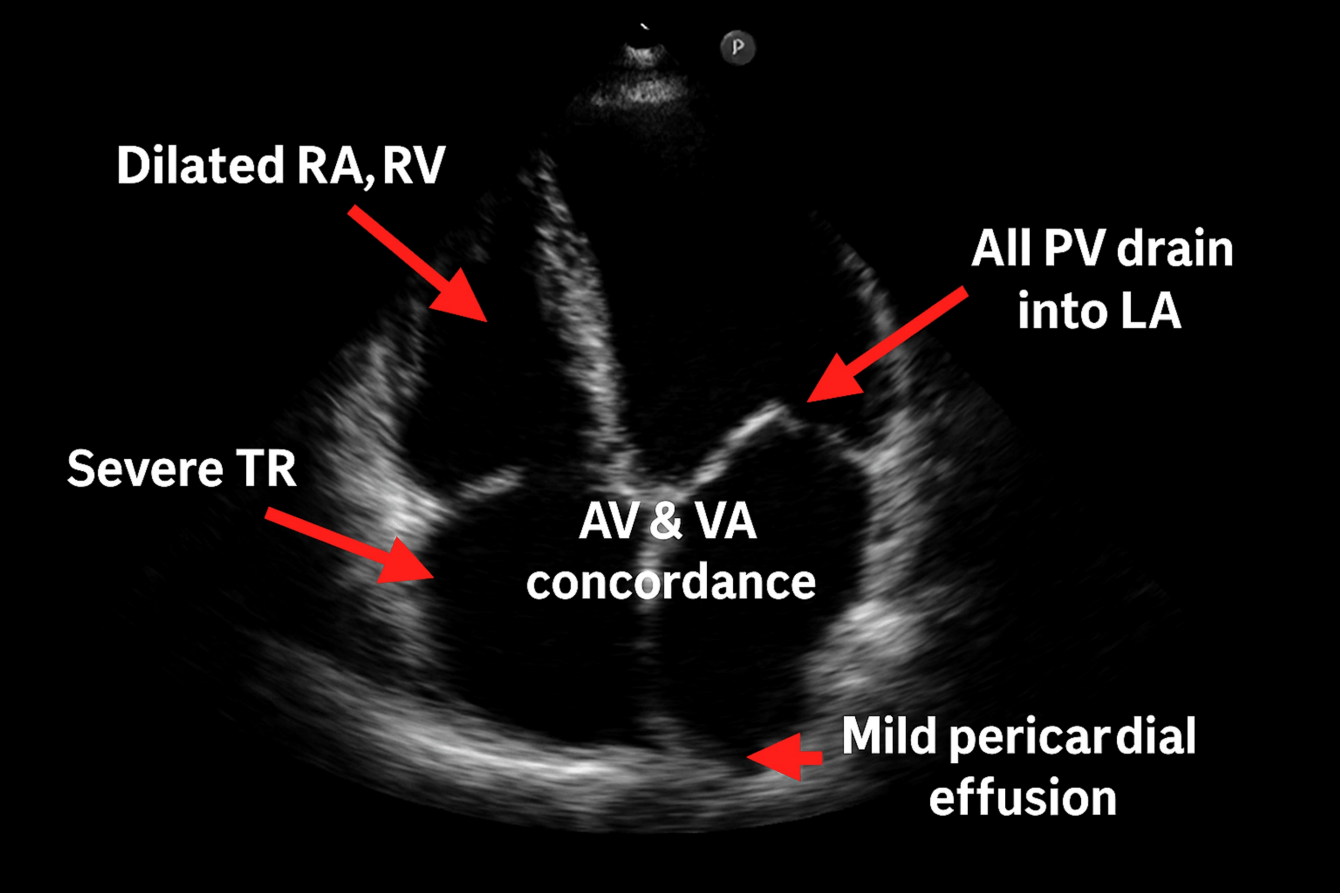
Echocardiography image of case 2 TR: Tricuspid regurgitation
